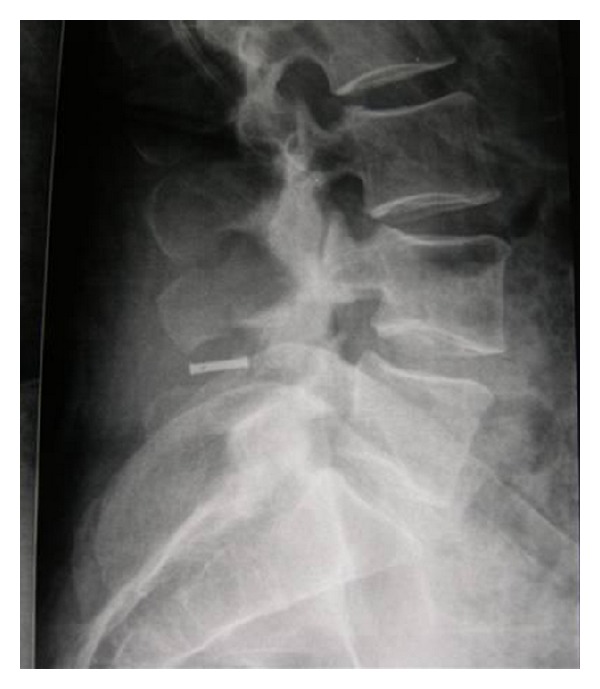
The lateral X-ray image reveals the radiographic marker of the BacJac implanted at L4-L5 interspinous area.
